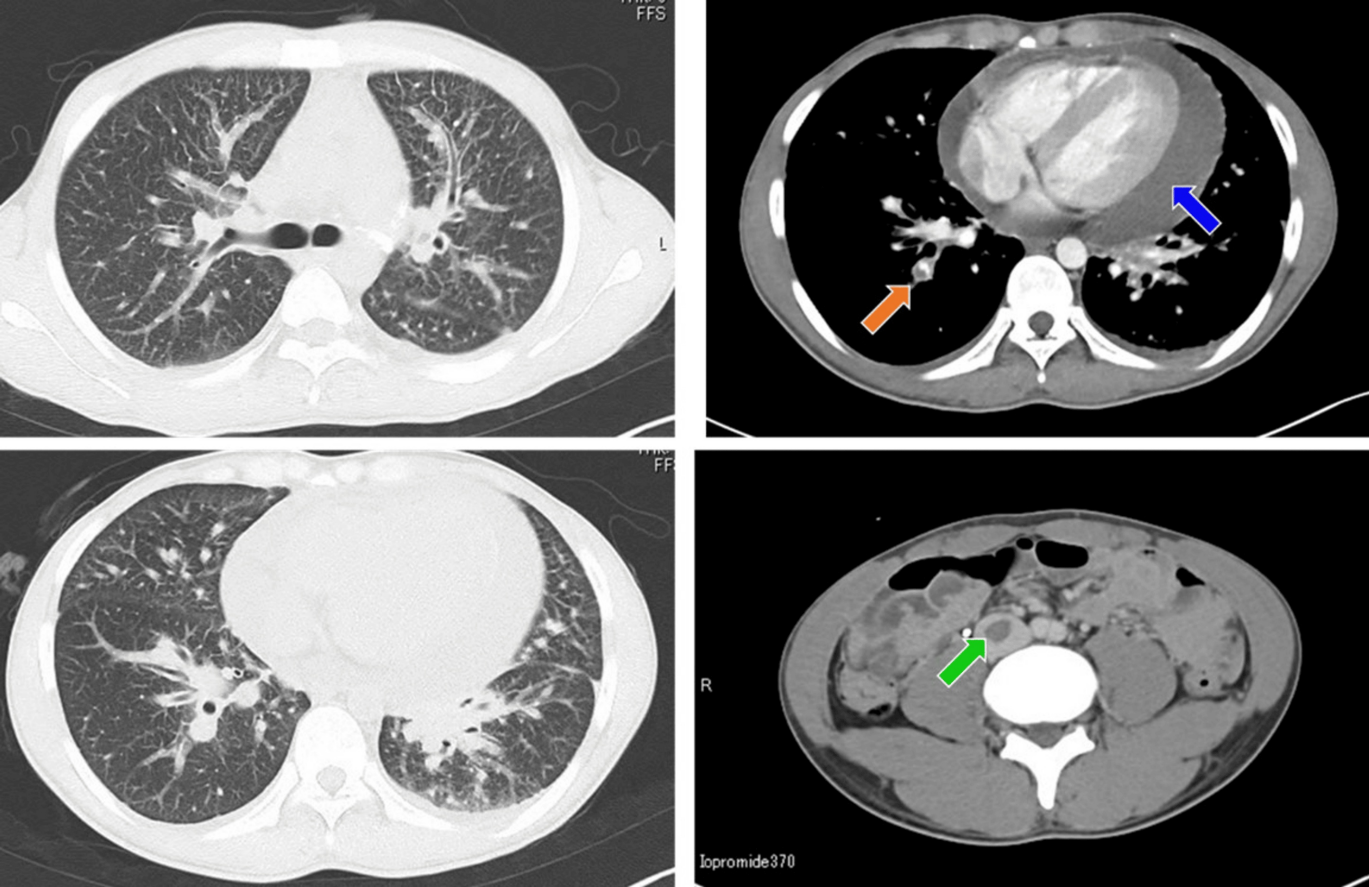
Chest CT Chest CT showing cardiac tamponade (blue arrow), pulmonary emboli (orange arrow), and deep vein thrombosis (green arrow). CT: computed tomography
